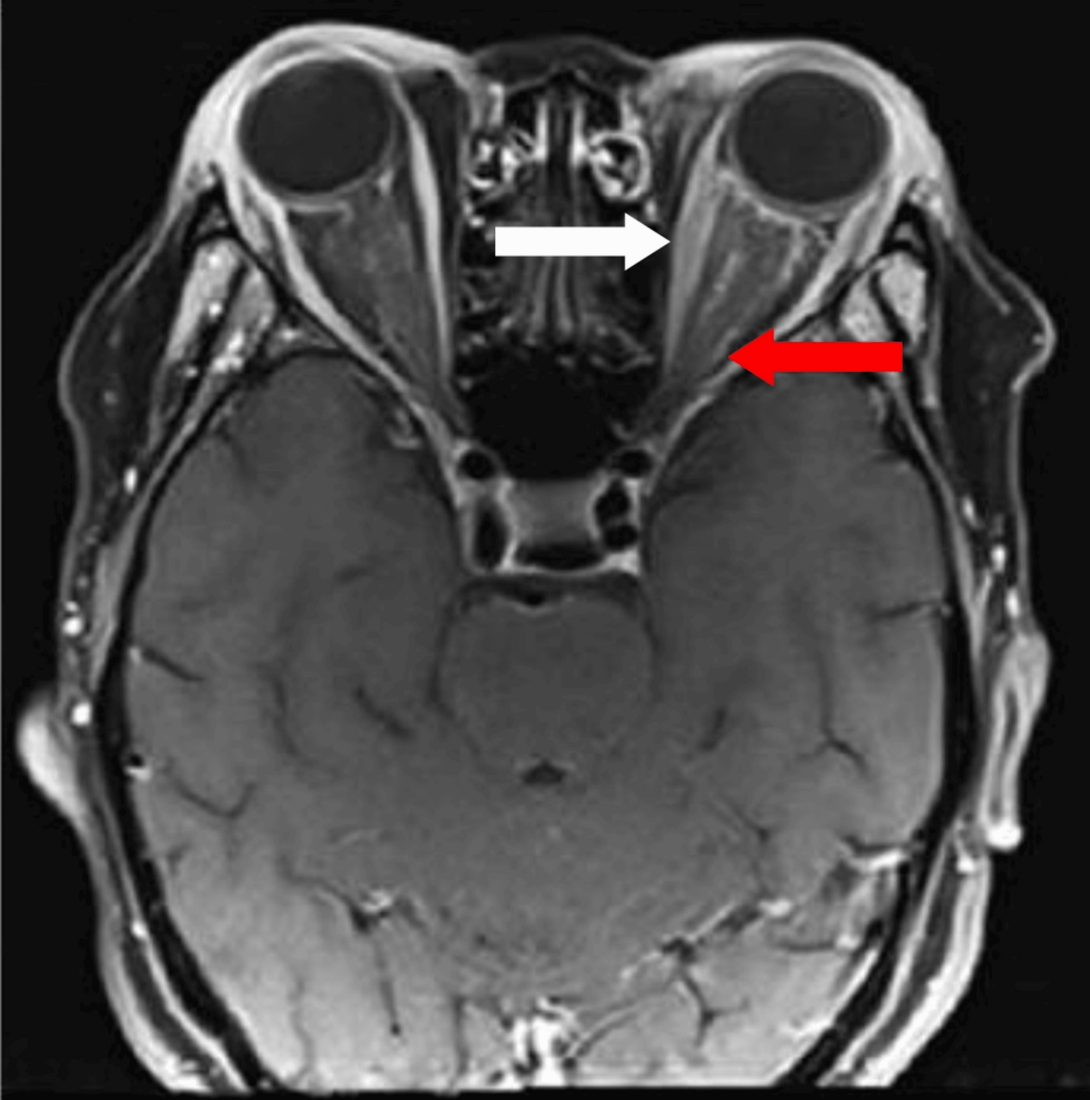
Gadolinium contrast MRI of the brain shows the bulky left optic nerve with perineural enhancement (red arrow) and bulky left medial rectus muscle (white arrow).
